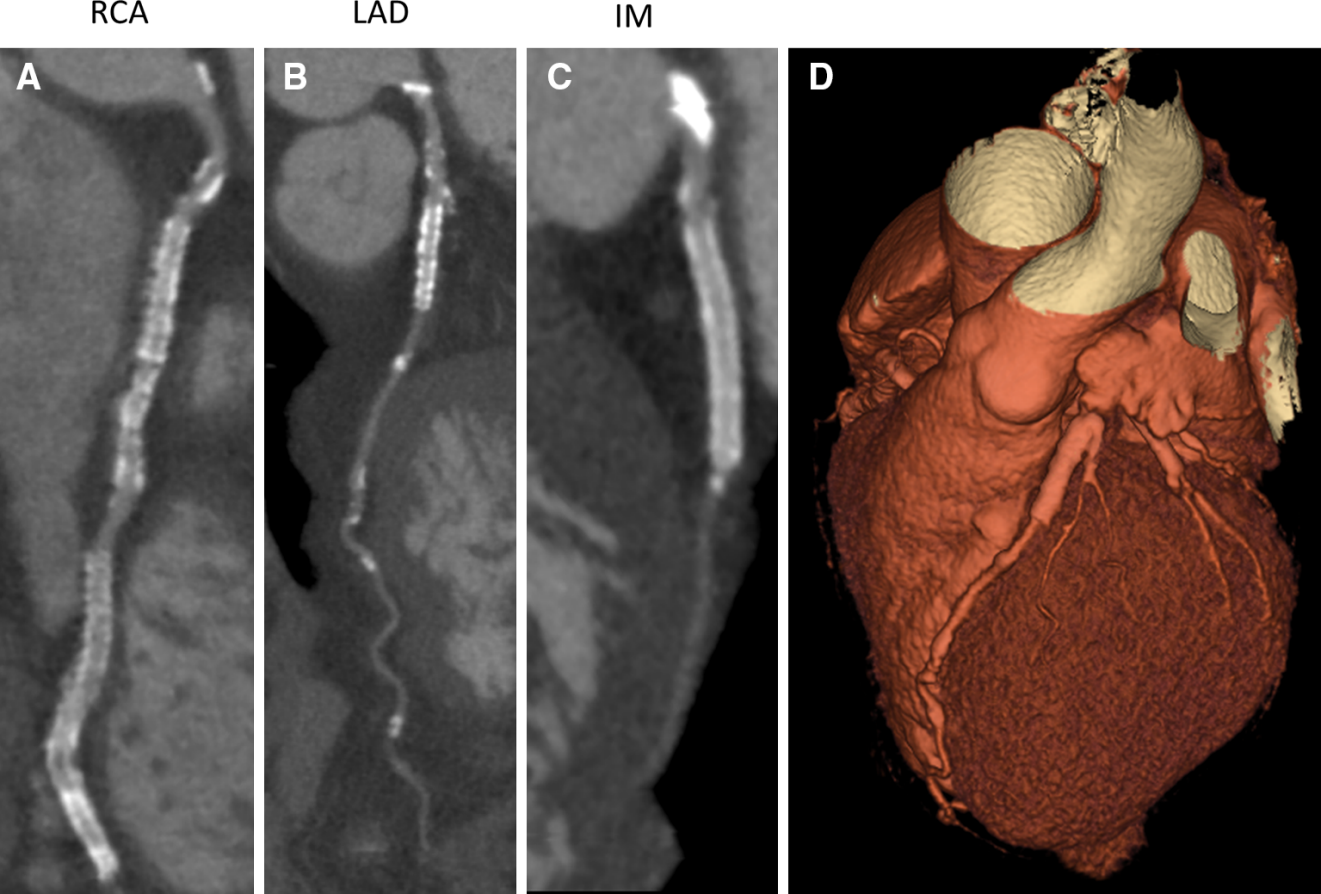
Coronary CTA depicting 2 stents in the RCA (A), 1 stent in the proximal LAD (B), and 1 stent in the IM (C). (D) 3D model of the heart and coronary arteries. *CTA*, coronary computed tomography angiography; *RCA*, right coronary artery; *LAD*, left ascending coronary artery; *IM*, intermediate branch
